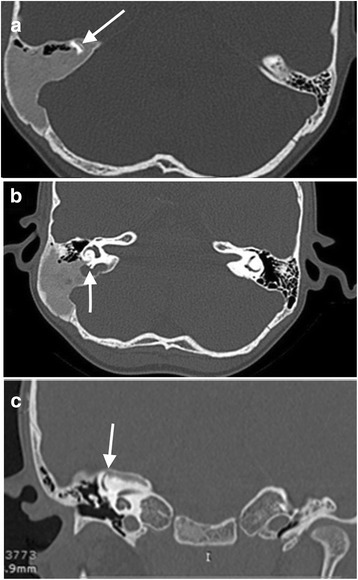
a Coronal CT scan shows involvement of the petrous part of the right temporal bone. The superior semicircular canal is surrounded by dysplasia. b Axial CT scan shows erosion of the labyrinth and involvement of the posterior semicircular canal. c Axial CT scan shows massive changes in the petrous part and a fistula of the superior semicircular canal
